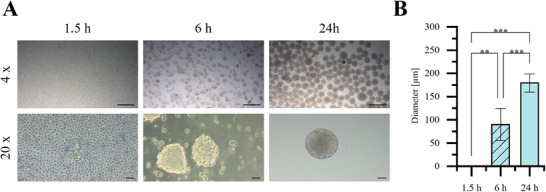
A) Appearance of EBs taken with different objective lenses. 4 x: Scale bar = 200 µm 20 x: Scale bar  =  50 µm. B) Distribution of EB diameter by increasing the duration of ultrasound exposure, 1.5 (no EBs have been found), 6 (n = 299), and 24 h (n = 136). Data are presented as mean ± SD (The *p*‐values were determined using one‐way ANOVA with a Tukey post hoc test, *p* < 0.05 (*), *p* < 0.01 (), and *p* < 0.001 (*), “n.s.”: no significant difference.).
